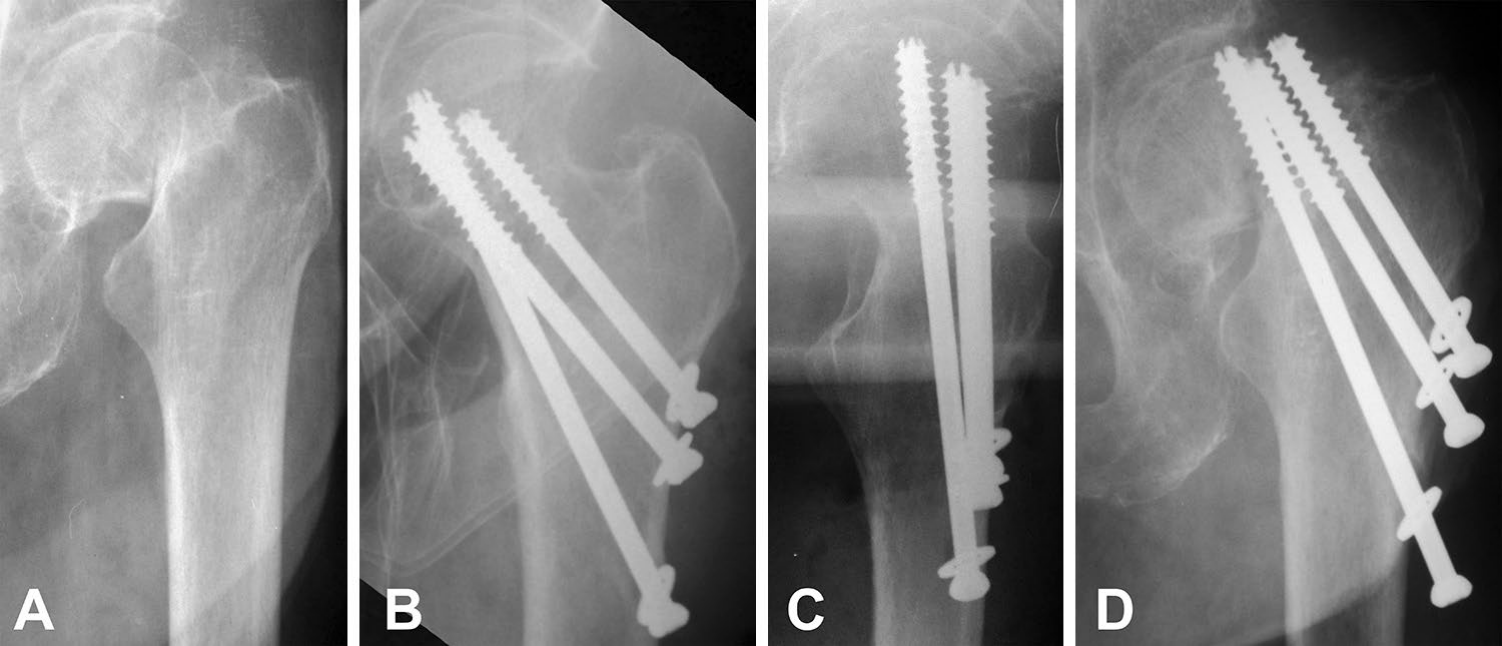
Exemplified X-rays of a 74 yo female patient with complete fixation failure (fragment redislocation) after BDSF treatment: AP view diagnostics (a), postoperative AP view (b), postoperative lateral view (c) and AP view 2-month follow-up (d). Lack of posterior cortical support and malreduction are seen on the postoperative X-rays (b, c)
